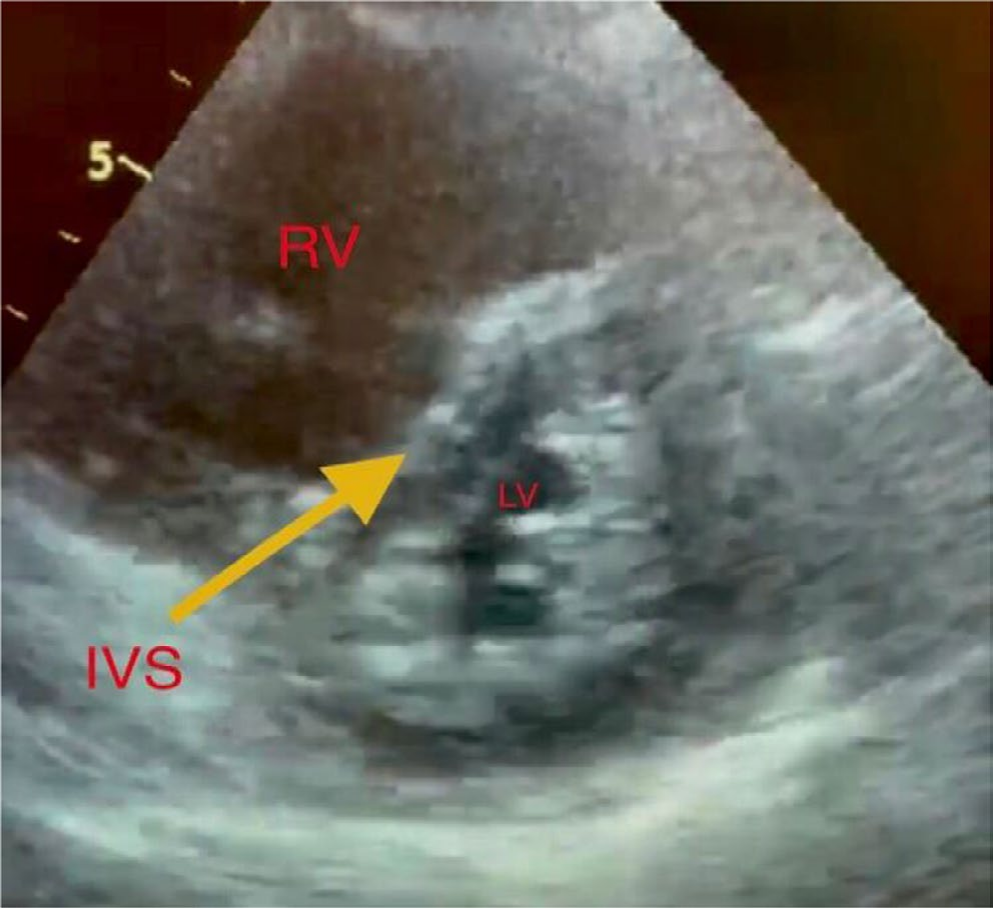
Parasternal short‐axis view showing dilated right ventricle (RV) with interventricular septum (IVS) shifted toward small left ventricle (LV)
